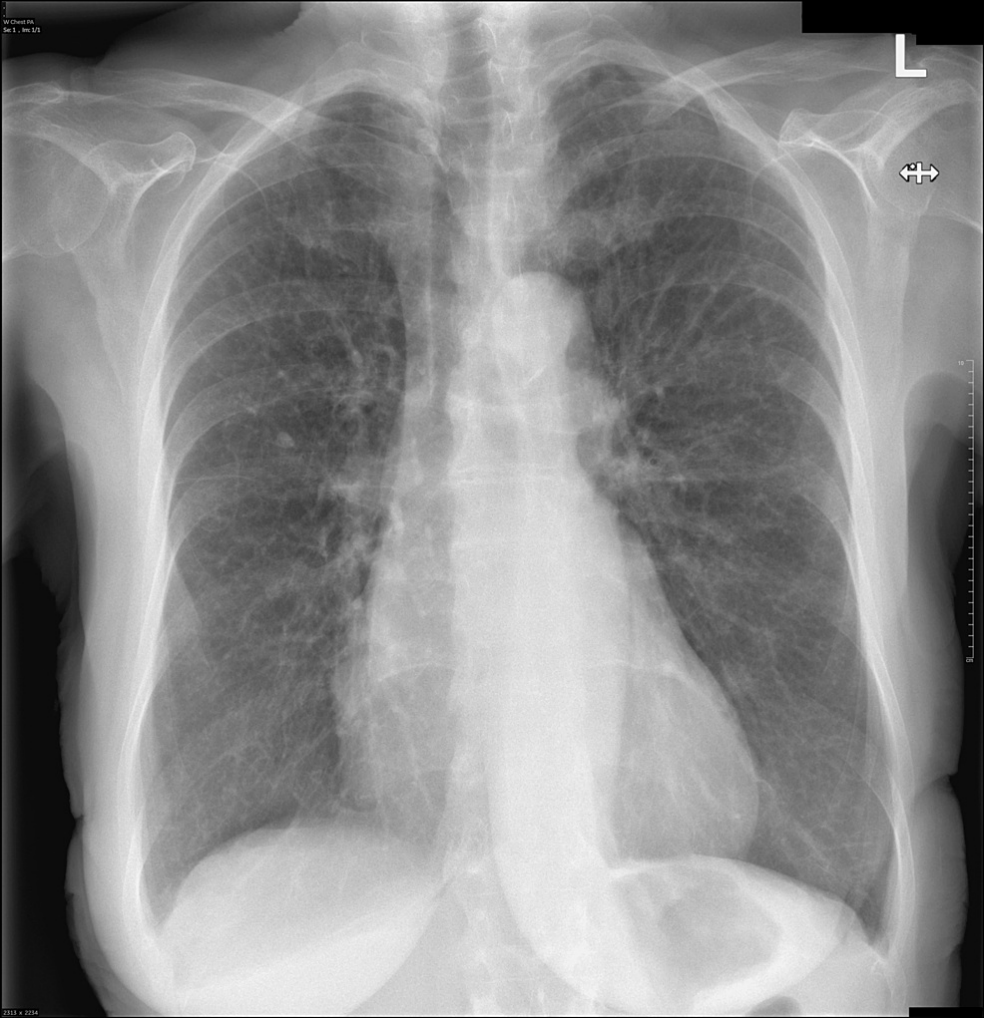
A posterior-anterior view of a chest radiograph. The heart is enlarged with a cardiothoracic ratio of 54%. The lungs are hyperinflated. No focal lung lesion, consolidation, or pleural effusions are identified.
